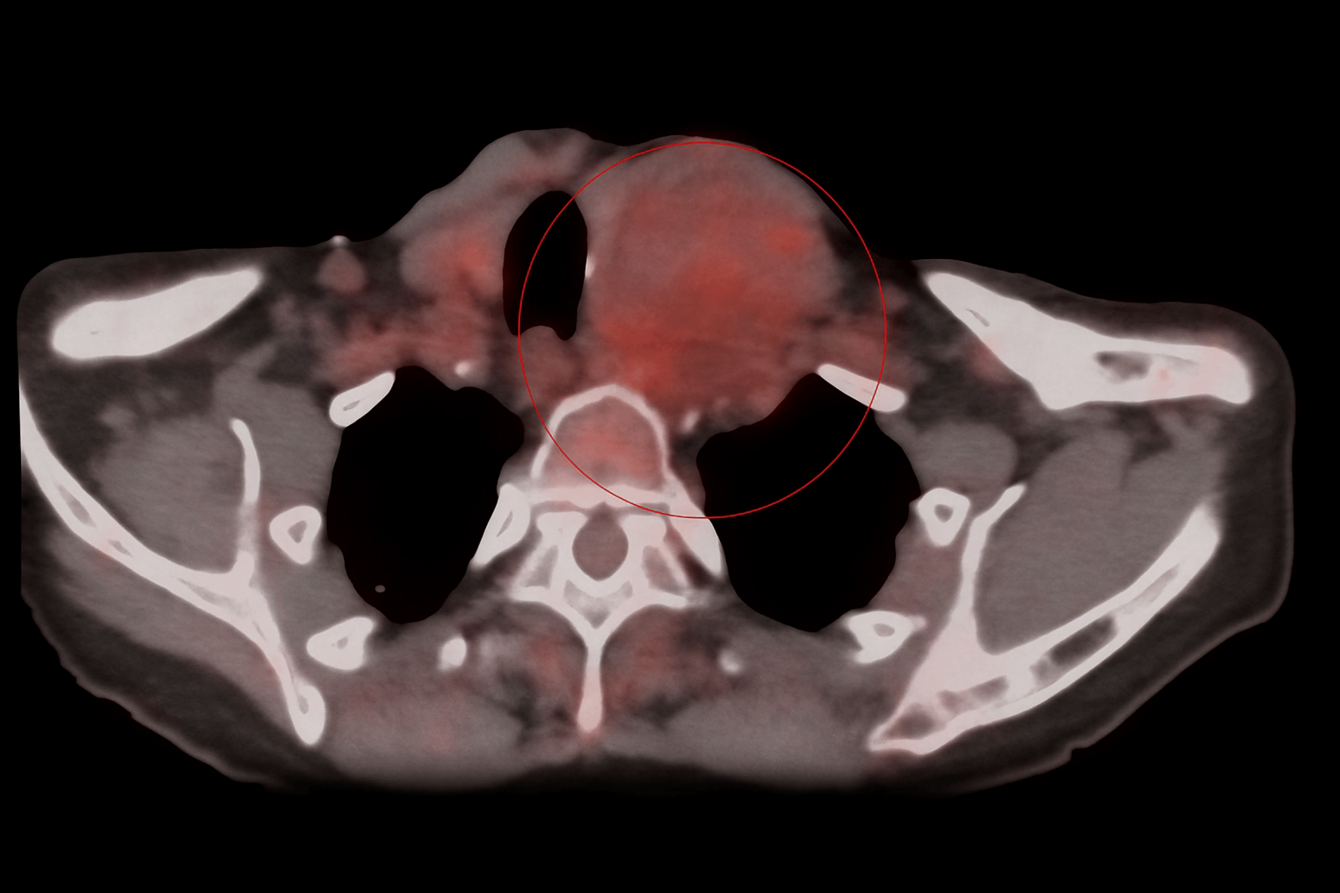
Axial PET-CT image of the neck demonstrating a soft tissue mass in the left lower cervical region with increased heterogeneous tracer uptake Scanned locally within Pilgrim Hospital, United Lincolnshire Teaching Hospitals NHS Trust PET-CT: positron emission tomography-computed tomography
